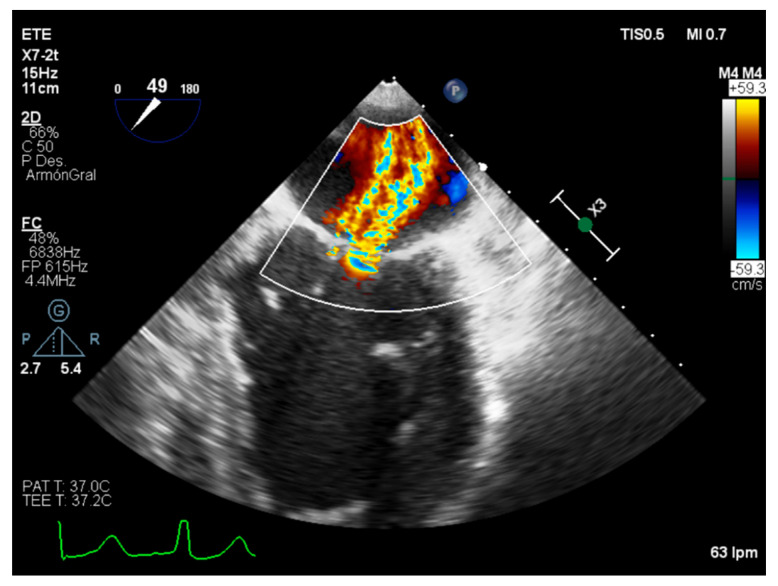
Transesophageal echocardiography. Mitral valve from Figure 4 with mild mitral regurgitation after transcatheter edge-to-edge repair procedure.
